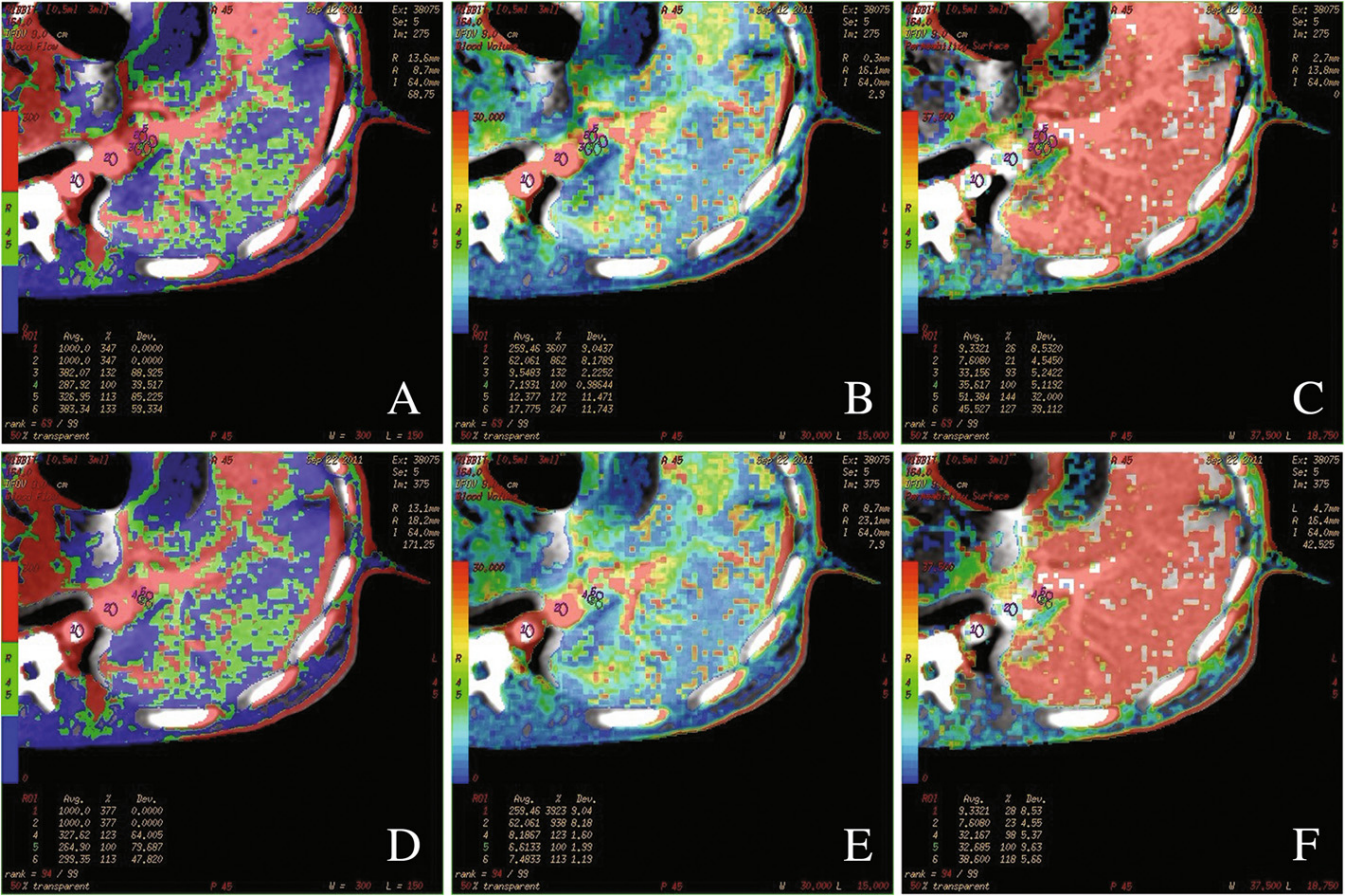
PVTT CT perfusion images before and after Endostar treatment. Note: PVTT Pre-treatment TBF (Figure 3-A), TBV (Figure 3-B), PS (Figure 3-C); PVTT post-treatment TBF (3-D), TBV (Figure 3-E), PS (Figure 3-F). Figure 3-A, 3-D tumor region demonstrated high blood perfusion state (red), Figure 3-B, 3-E tumor region showed high blood perfusion state (red), Figure 3-C, 3-F tumor region demonstrated high blood perfusion state (red). Post-treatment perfusion parameters were significantly lower than those at baseline. There was no notable change on tumor size.
